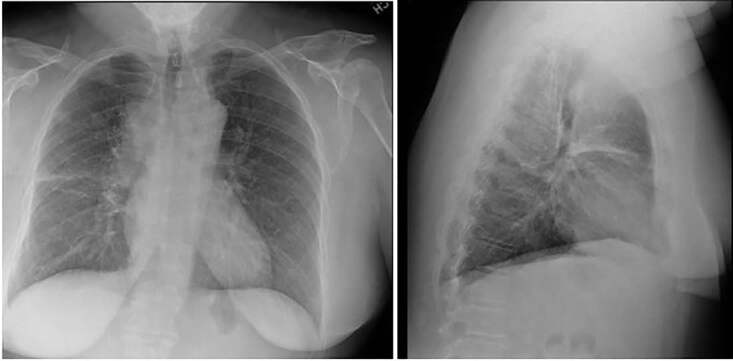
Posterior-anterior and lateral chest radiograph of a 54-year-old female with facial swelling.
